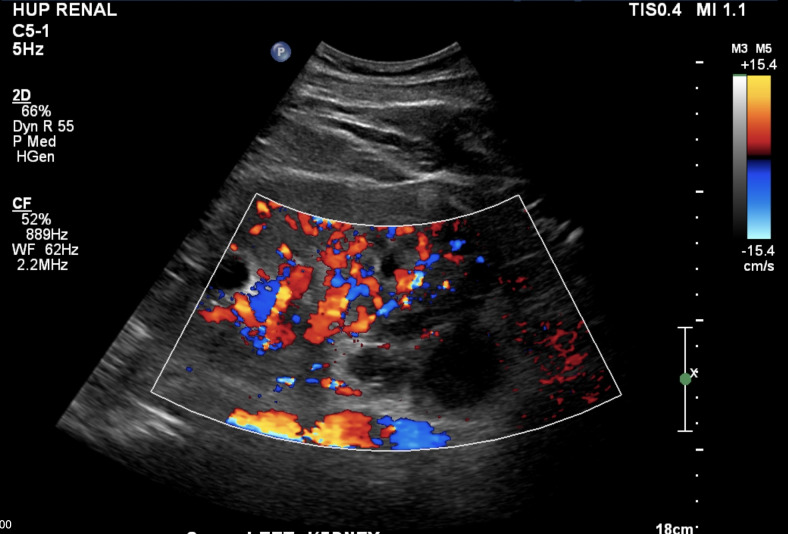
Doppler imaging of left kidney in long axis demonstrating lack of fast flow in the area of suspected plasmacytomas.
